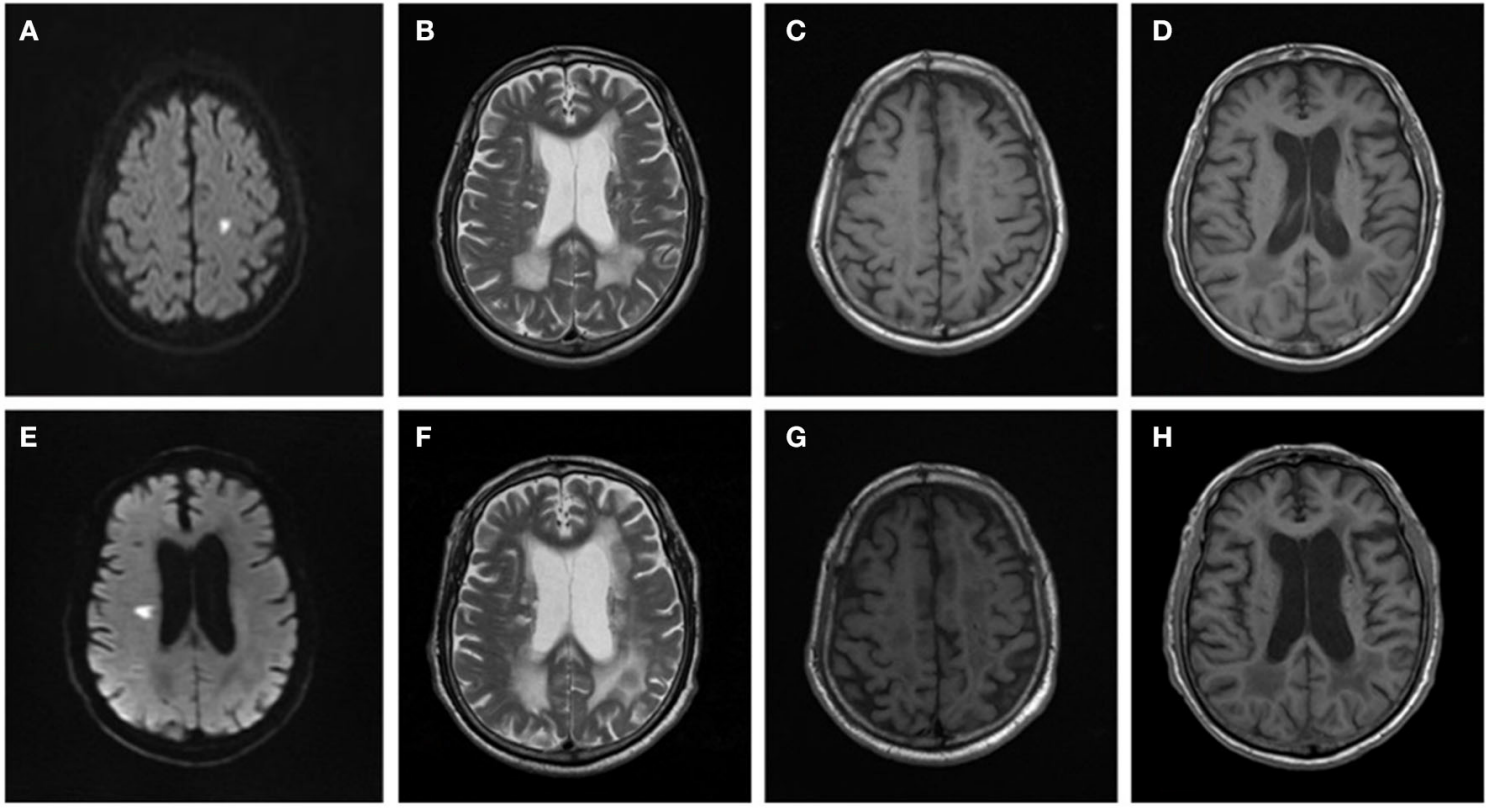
Brain MRI changes in a patient with arteriolosclerosis CSVD. Male, 66 years old, was admitted to the hospital due to right limb weakness for 2 days in 2014. Brain MRI: (A) DWI showed a small flake of high signal in the left centrum semiovale. (B) T2WI showed irregular hyperintensity near the ventricle. (C, D) T1WI showed enlargement of the sulci and slight enlargement of the ventricles. In 2018, the patient was readmitted to the hospital due to dysarthria and left limb weakness for 1 day. Brain MRI: (E) DWI showed a small flake of high signal near the right lateral ventricle. (F) T2WI, the range of irregular hyperintensity near the ventricle was enlarged and extended to deep white matter with large area fusion. (G, H) T1WI showed enlargement of the sulci and enlargement of the ventricles, which made progress compared with 2014.
